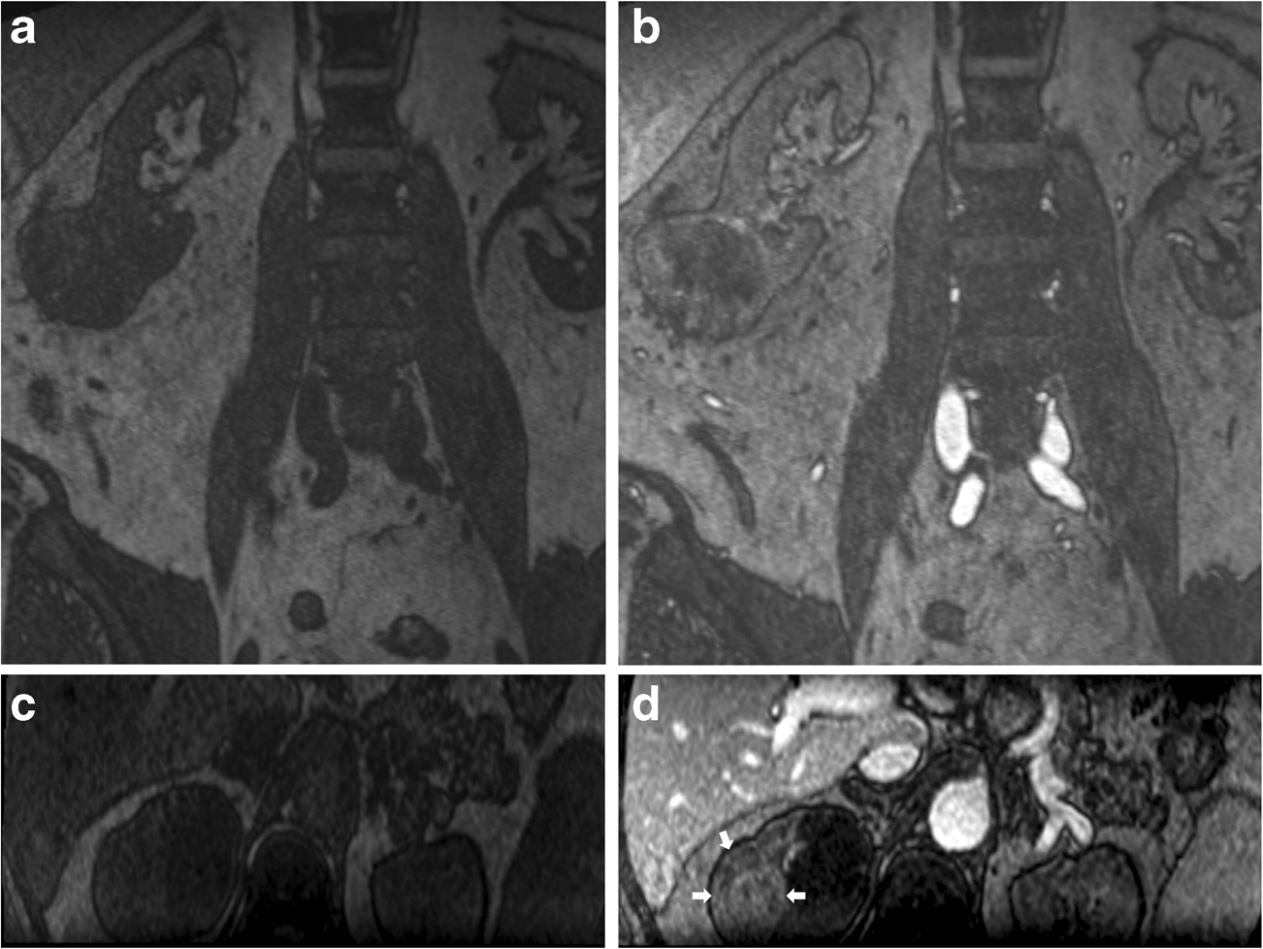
Ferumoxytol-enhanced magnetic resonance angiography (FeMRA) showing incidental renal tumours in two patients. (A) Coronal pre- and (B) post-contrast T1-weighted first-pass acquisitions through the upper abdomen showing tumour with peripheral enhancement and central necrosis originating from the lower pole of the right kidney. (C) Transverse pre- and (D) post-contrast T1-weighted first-pass acquisitions through the upper abdomen from another patient showing a large partially enhancing right renal mass (arrows)
